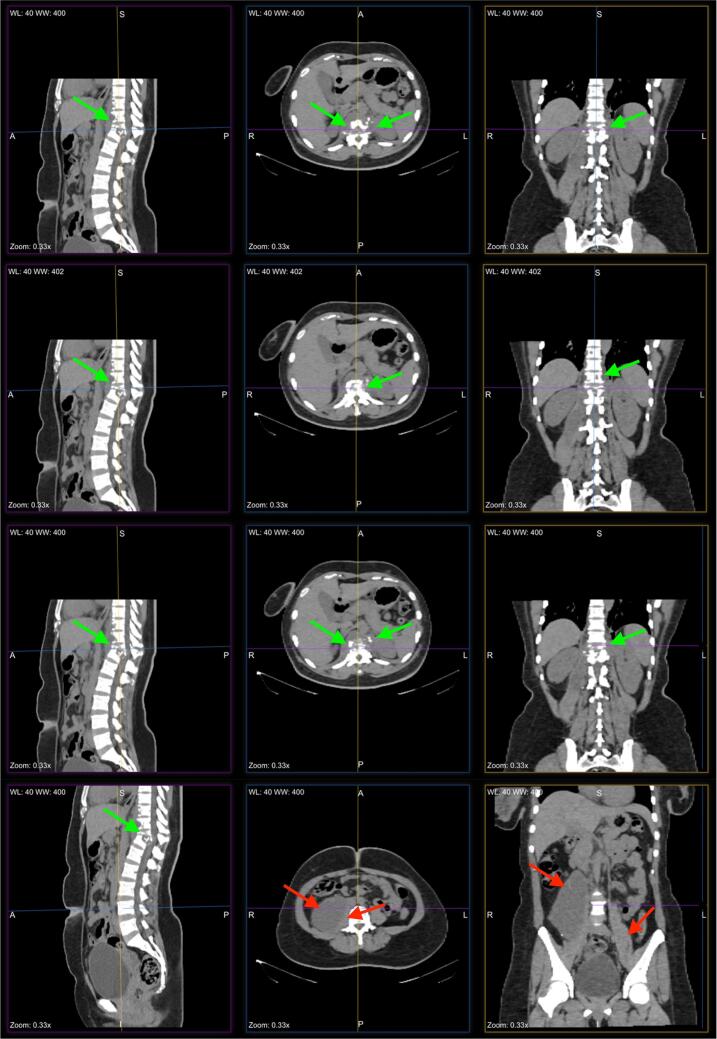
Computerized tomography scan of female patient showing bony destruction of the T8-T12 vertebrae as well as loss of height and kyphosis of T11. A paravertebral abscess can be seen extending from T8 to L1 level. The right psoas abscess is more easily appreciated on CT due to its large size (5 × 4.3 × 15.3 cm) as compared to left-side psoas abscess (1.1 × 1.3 × 3.4 cm). Key features including bilateral psoas abscesses and bony destruction of the spinal column have been identified with red and green arrows respectively. (For interpretation of the references to colour in this figure legend, the reader is referred to the web version of this article.)
